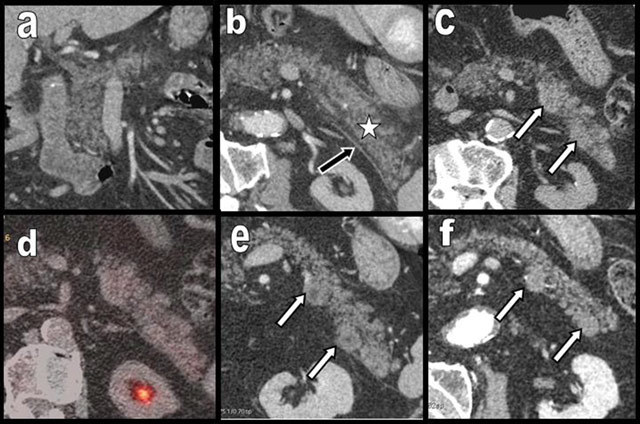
Coronal cephalic (a) and axial oblique caudal views (b) show subtle signs of acute caudal pancreatitis superimposing on diffuse FI of a 75-year-old patient (type 2b) suffering from colonic carcinoma. Discrete edema is found between the glandular lobules (white star), and a small linear effusion (black arrow) is found in the anterior pararenal fascia. Follow-up studies – unenhanced CT at day 5 (b) and contrast enhanced CT at day 30 (e) – show inflammatory agglutination of lobules mimicking pseudotumoral nodules (white arrows). Fortunately intercurrent 18F-FDG PET/CT, performed in the follow-up of colonic carcinoma at day 15 showed negative FDG uptake (d). Slow regression of the necrotizing pseudotumoral nodules is clearly visible after 10 months (f).
